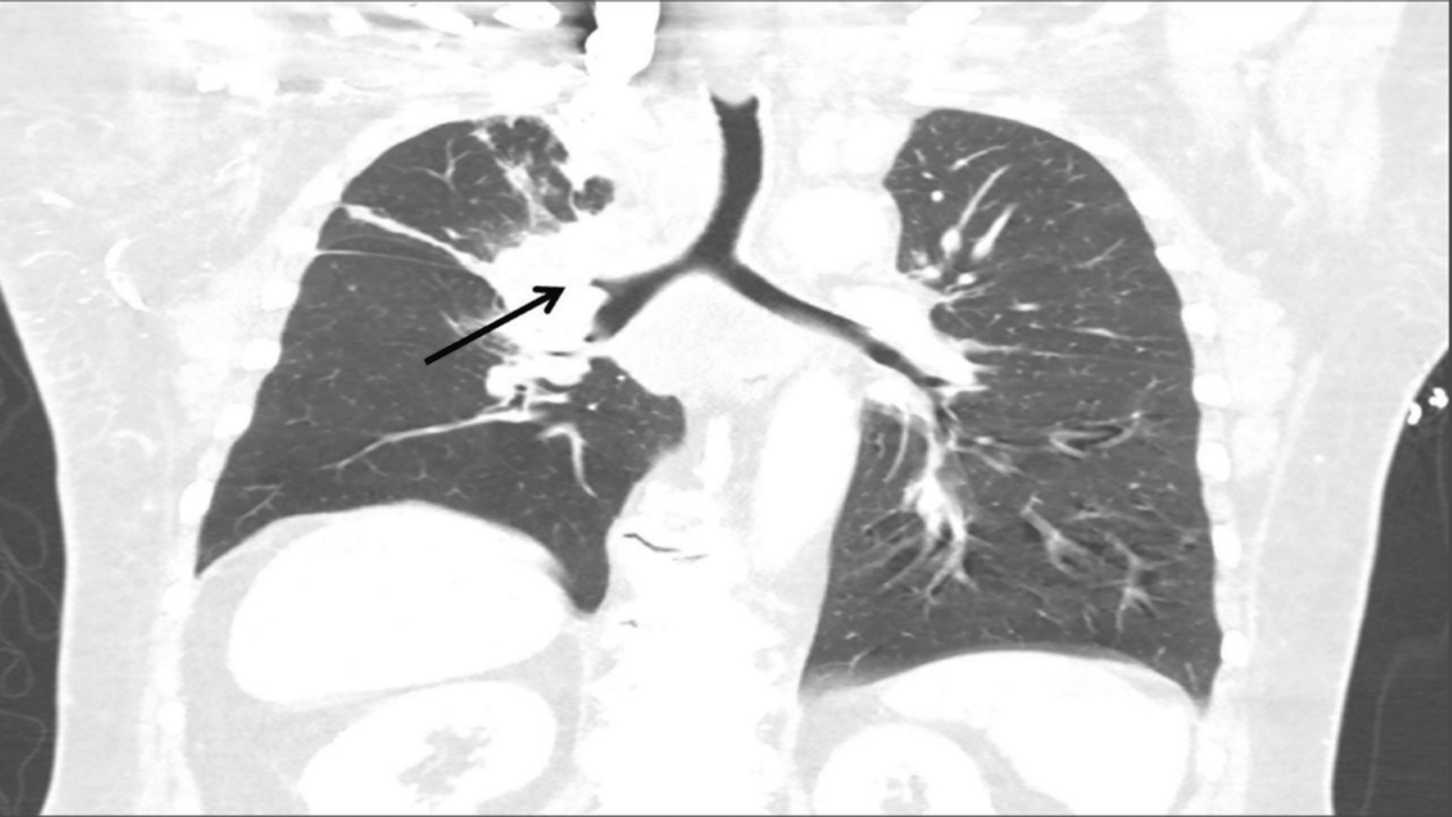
Chest CT scan (coronal view) showing compression of the right upper lobe bronchus
